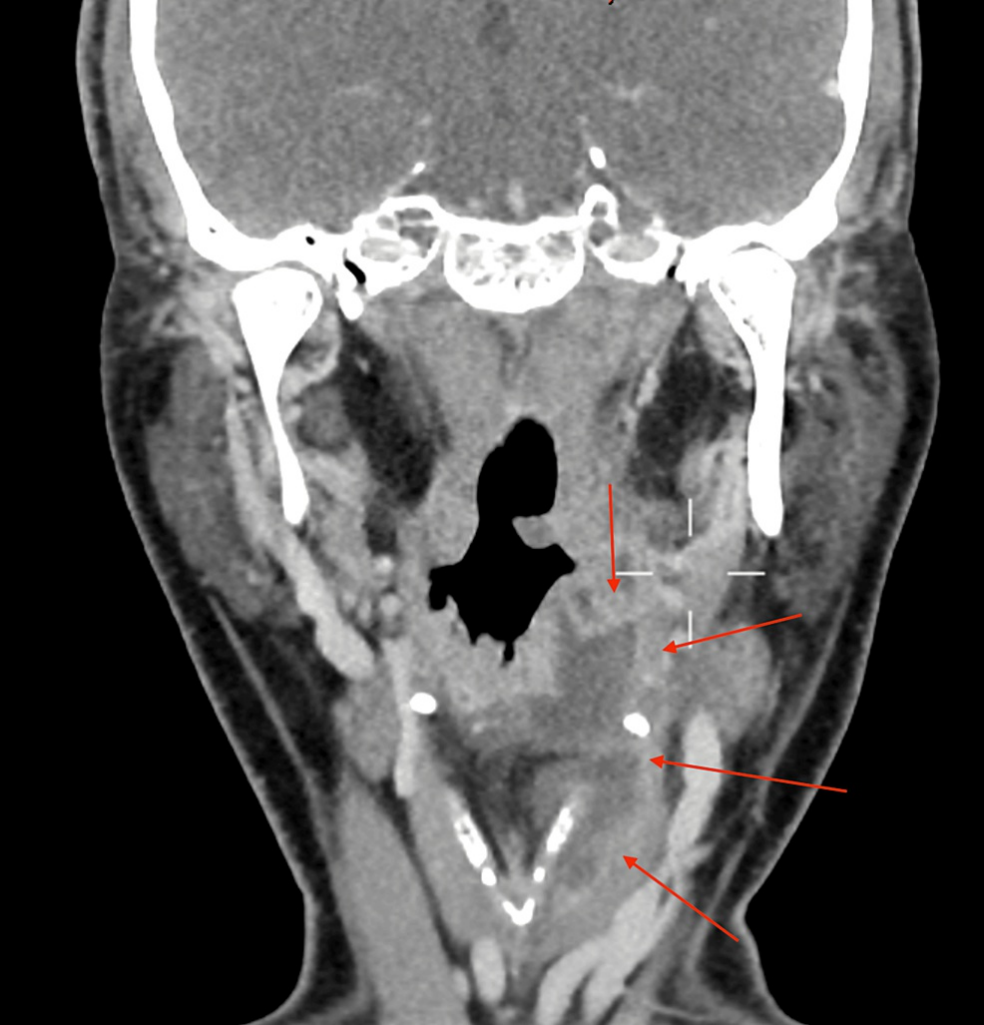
Coronal view of a CT scan of the neck demonstrating a left-sided collection extending from the glottis to the thyroid cartilage and between the strap muscles (red arrows)
